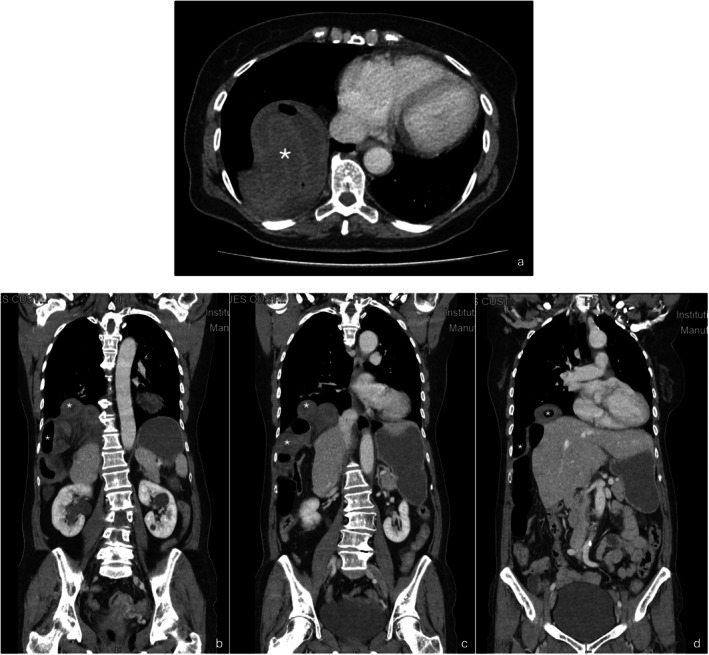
CT scan—transverse (a) and sagittal views (b, c, d) showing the bowel segments passing laterally to the liver and into the thoracic cavity (marked as *).
